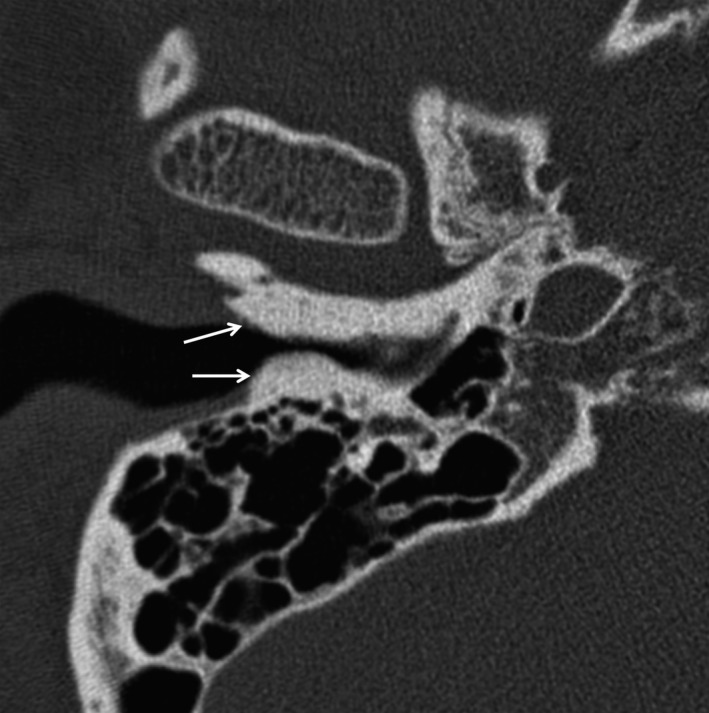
Computed tomography image of right temporal region.
